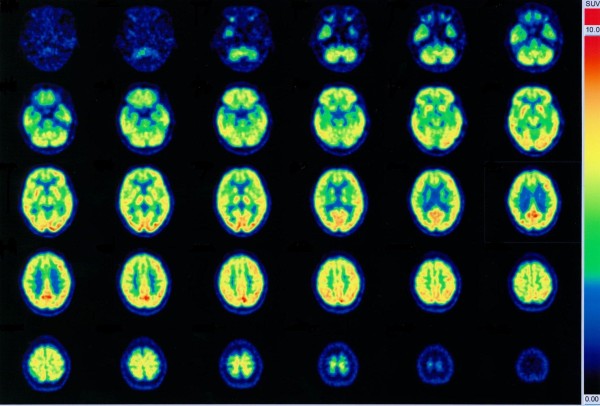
The FDG PET scans of the patient. Bilateral frontal lobe hypometabolism was noted.
